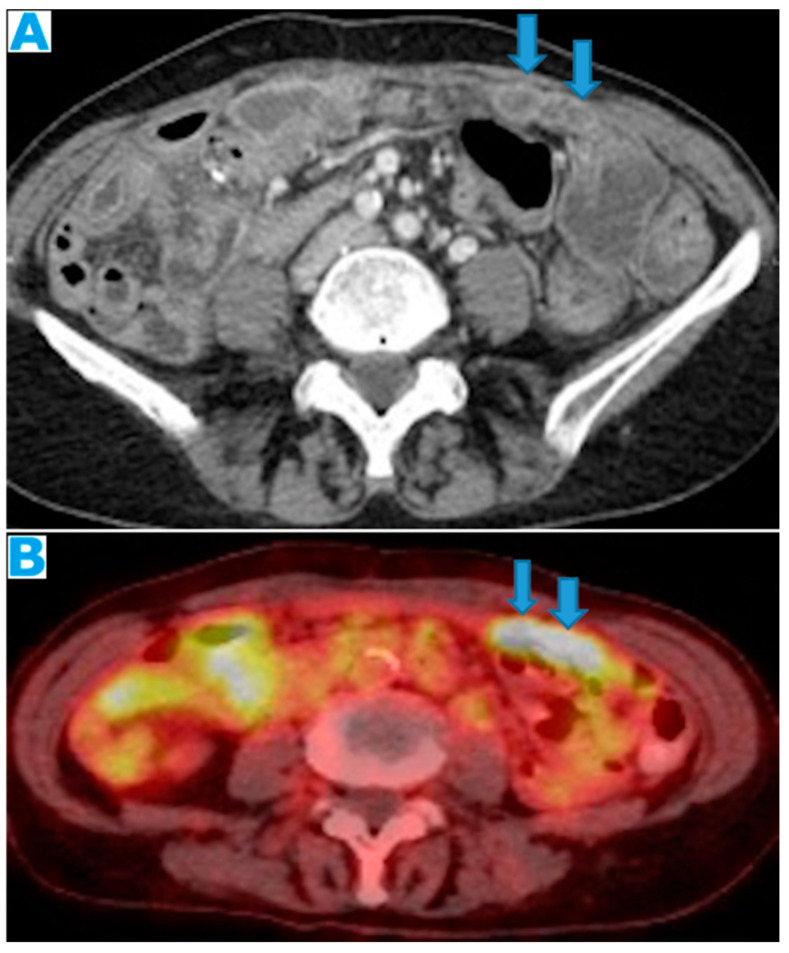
Axial CE-CT (A); FDG-PET-CT (B). PC from colon adenocarcinoma: nodular deposits within the omentum (arrows) that were originally mistaken for SB loops upon CT due to the scarce intrabdominal fat.
